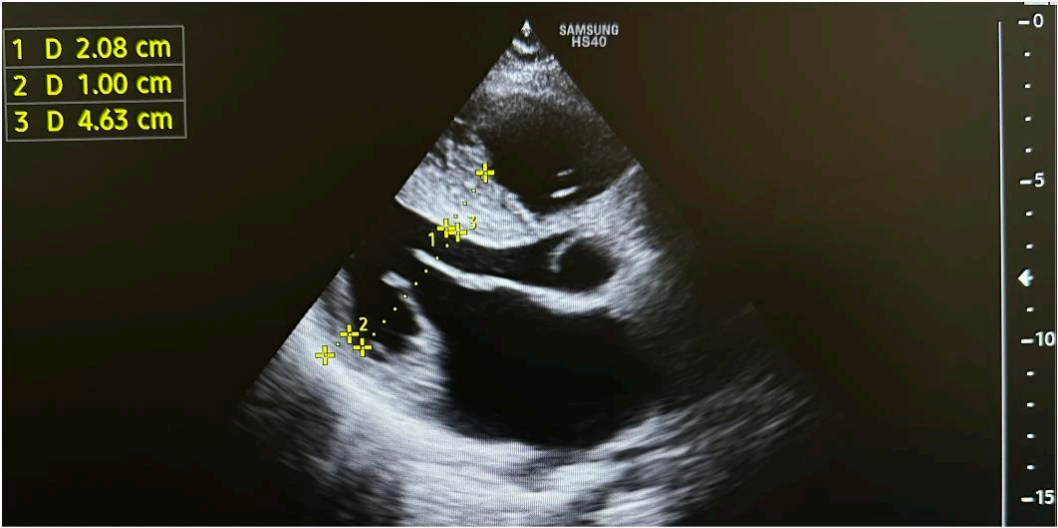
After the completion of the two ethanol ablation procedures, a subsequent echocardiography revealed an interventricular wall thickness of 21 mm and a gradient of 7 mmHg at the LVOT.
